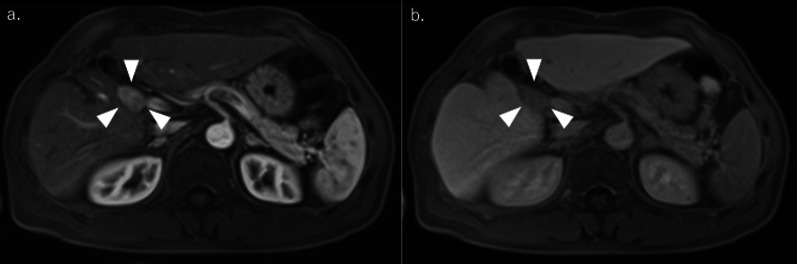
Contrast-enhanced MRI. (a early phase, b delayed phase) similarly shows a mass lesion at the hepatic hilum (white arrow)
